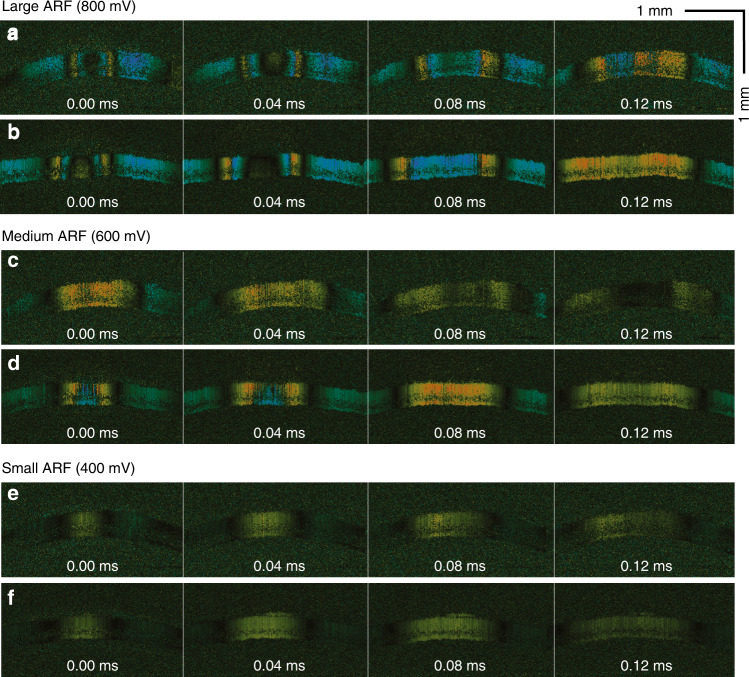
Time-lapse Doppler OCT B-scans from SS-OCE_COV_ and SS-OCE_CP_ systems with different ARFs. a, c, e Time-lapse Doppler OCT B-scans from the SS-OCE_COV_ system with large, medium, and small ARFs, respectively. b, d, f Time-lapse Doppler OCT B-scans from the SS-OCE_CP_ system with large, medium, and small ARFs, respectively
